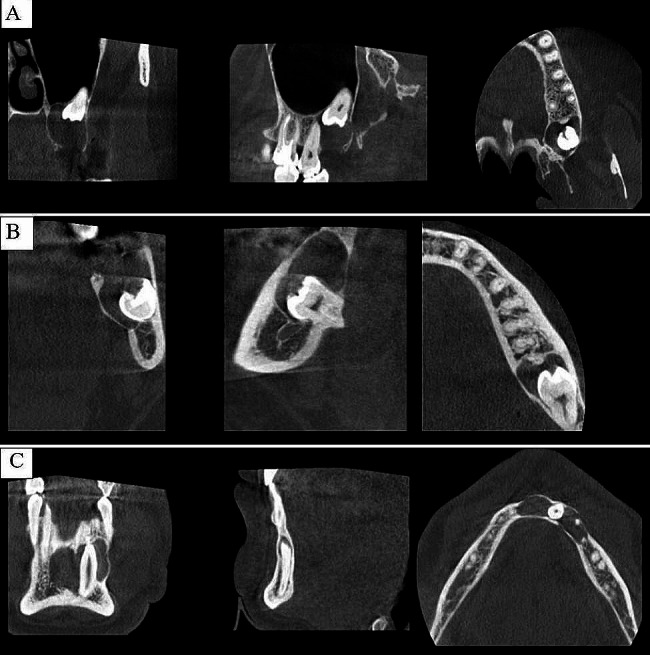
CBCT images of (A) Central, (B) Lateral, and (C) Circumferential Dentigerous Cysts in coronal, sagittal and axial planes
